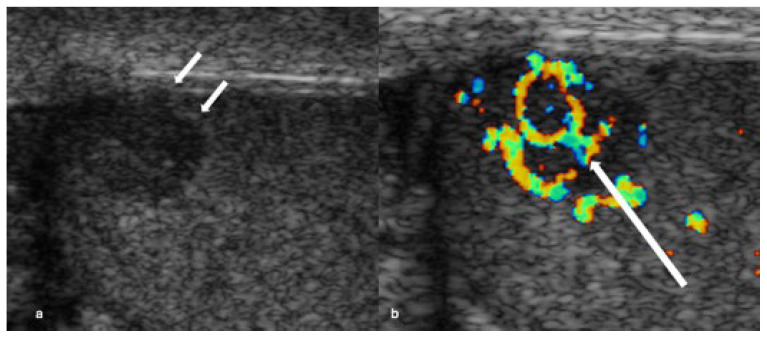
Benign Leydig cell tumour with a criss-cross intralesional vascular pattern. Longitudinal images of a benign Leydig cell tumour in the left testis: (a) grey-scale ultrasound shows a well-circumscribed hypoechoic lesion (8.0 × 5.0 × 5.0 mm; short arrows); (b) colour Doppler ultrasound demonstrates a criss-cross intralesional vascular pattern (long arrow), which can mimic appearances seen in malignant neoplastic lesions.
